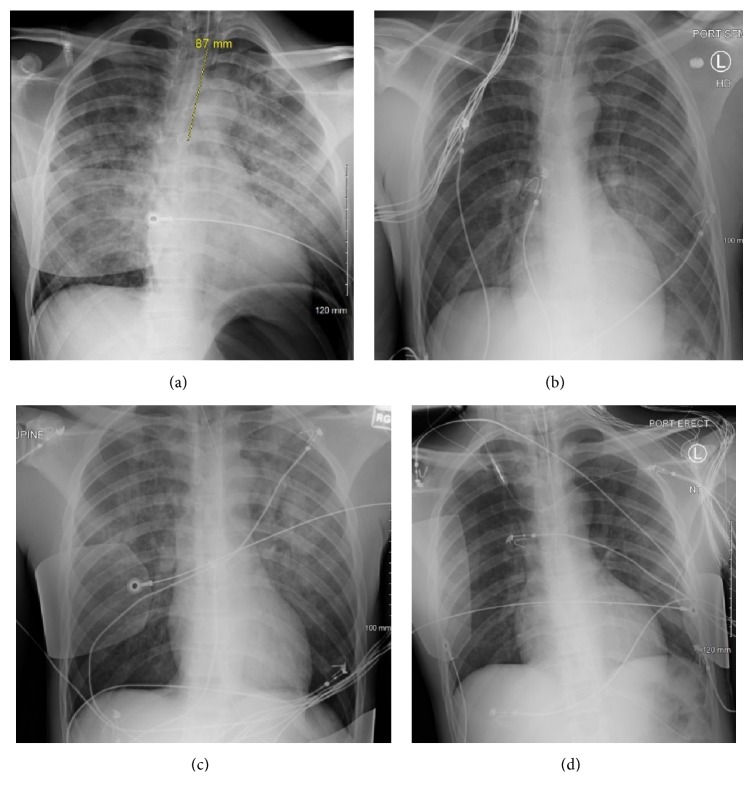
Chest radiographs from each admission. Initial postintubation radiograph (a) from his first admission showing diffuse bilateral infiltrates; repeat radiograph (b) showing resolution approximately 18 hours later. Initial radiograph (c) from the second admission again demonstrating bilateral opacities which resolved (d) within 48 hours.
